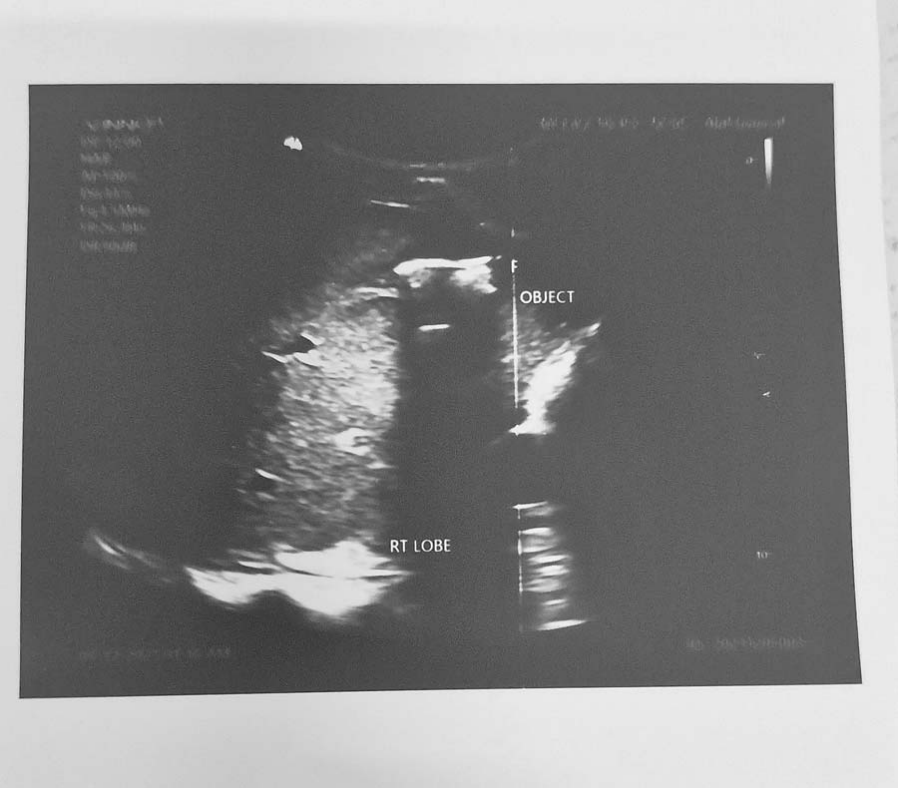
Abdominal ultrasound showing an object with an acoustic shade inside the liver parenchyma.
